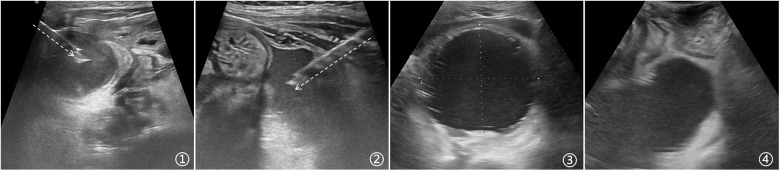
Appendiceal abscesses at different locations and the selection of puncture sites. (1) A single abscess cavity around the appendix in the right lower abdomen can be drained by inserting a needle for pus aspiration from the right lower abdomen, with the needle path visible along the arrow direction. (2) If the abscess is located in the left lower abdomen, ultrasound can be used to locate and select an appropriate entry point in the left lower abdomen for needle insertion. (3) The abscess is located behind the bladder, and under ultrasound, the bladder appears non-distended while the abscess is relatively large. (4) For an abscess located anterior to the rectum, this type of appendiceal abscess can be punctured via the perineal approach.
